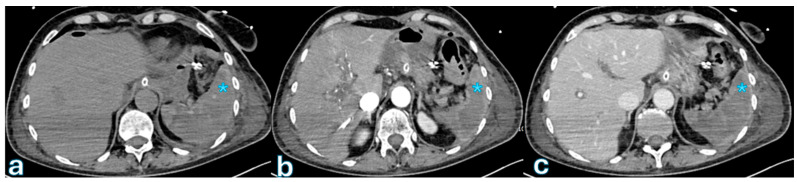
Non-contrast CT image (a) and post-contrast CT images with iodinated contrast in the arterial (b) and portal (c) phases, showing marked and persistent mottled opacification of the splenic artery, with subsequent lack of opacification in the downstream parenchyma (blue asterisk). This finding was observed in a patient who underwent major pancreatic resection for adenocarcinoma of the body of the pancreas one week prior and is consistent with embolic occlusion of the splenic artery, resulting in splenic infarction.
